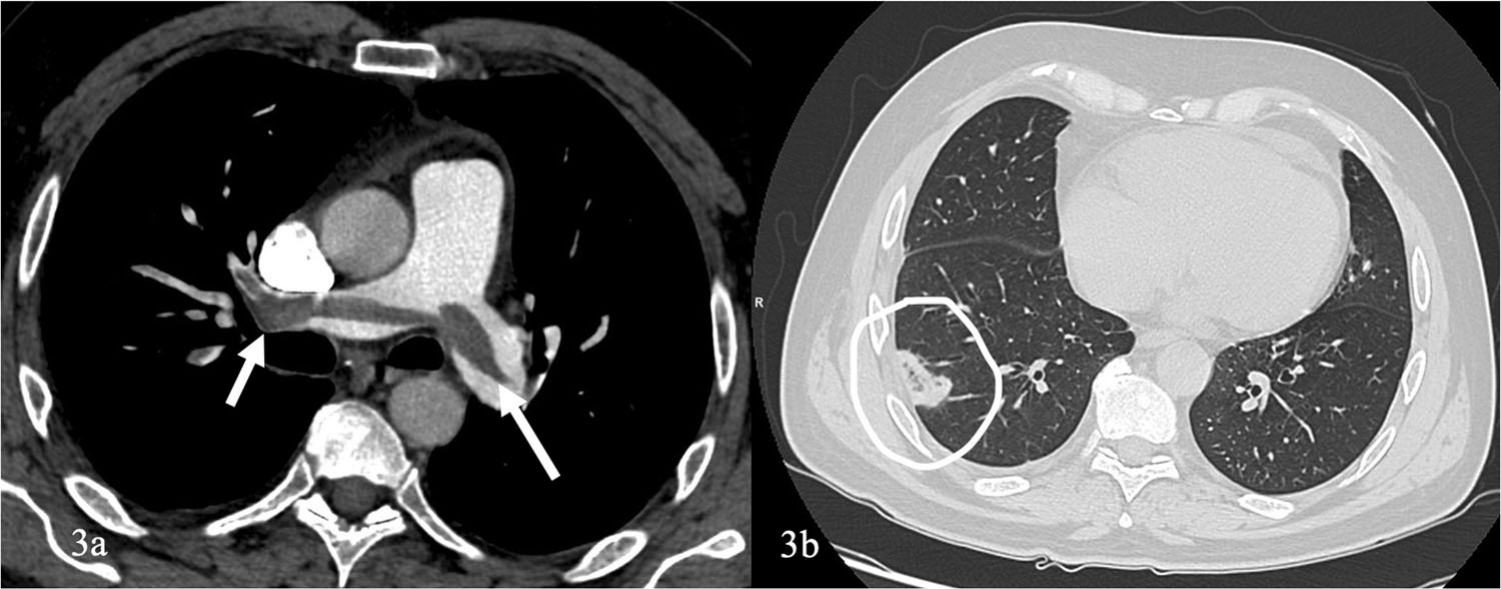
a Computed tomography angiogram scan of a 73-year-old that presented to the emergency department with deep vein thrombosis, hypotensive and with a drop of oxygen saturation, that confirms extensive bilateral pulmonary thrombus (arrows). b Pulmonary infarct is revealed in the right lower lobe (white circle)
